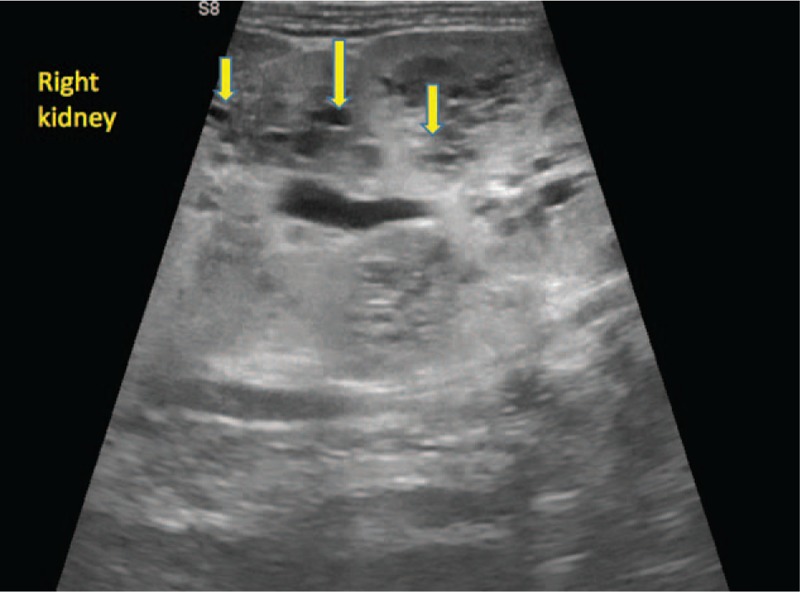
The ultrasound aspect of cystic lesions in the right kidney at the time of diagnosis.
